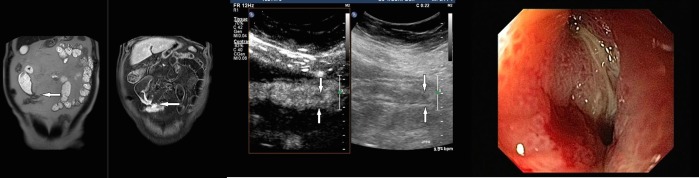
Forty- two-year-old male patient with Crohn’s disease and enteroenteral fistula of the terminal ileum. A: Coronal MR Enterography images (T2-weighted single-shot turbo spin-echo, on the left image; T1-weighted fat saturated, spectrally attenuated inversion recovery after Gadoteric acid administration, on the right image) of a patient with enteroenteral fistula and increased enhancement after contrast administration (white arrow). B: Longitudinal Contrast enhanced ultrasound of the affected ileum of the same patient (Gray scale ultrasound image on the right side; Contrast enhanced ultrasound image on the left side), showing severe wall thickening on the Gray scale ultrasound (white arrow) and strong enhancement after Sonovue administration on the Contrast enhanced ultrasound (white arrow), but no fistula. C: Endoscopic image of the same patient, with a fistula opening in the terminal ileum.
